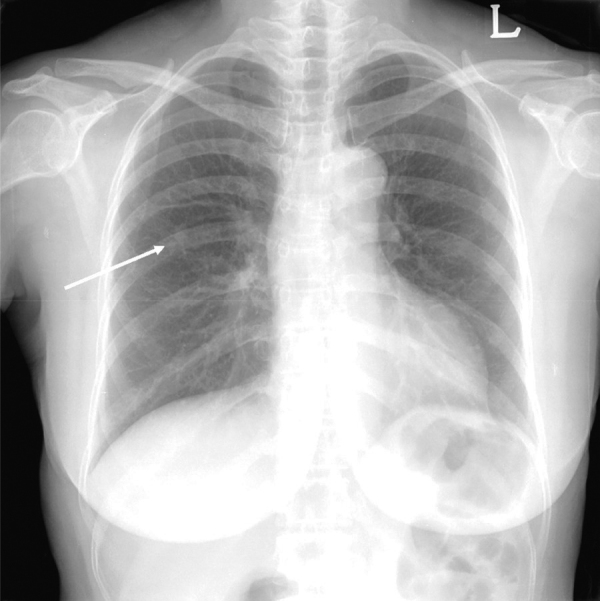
Initial chest radiograph shows an incidental nodule in the right upper lung field (arrow).
